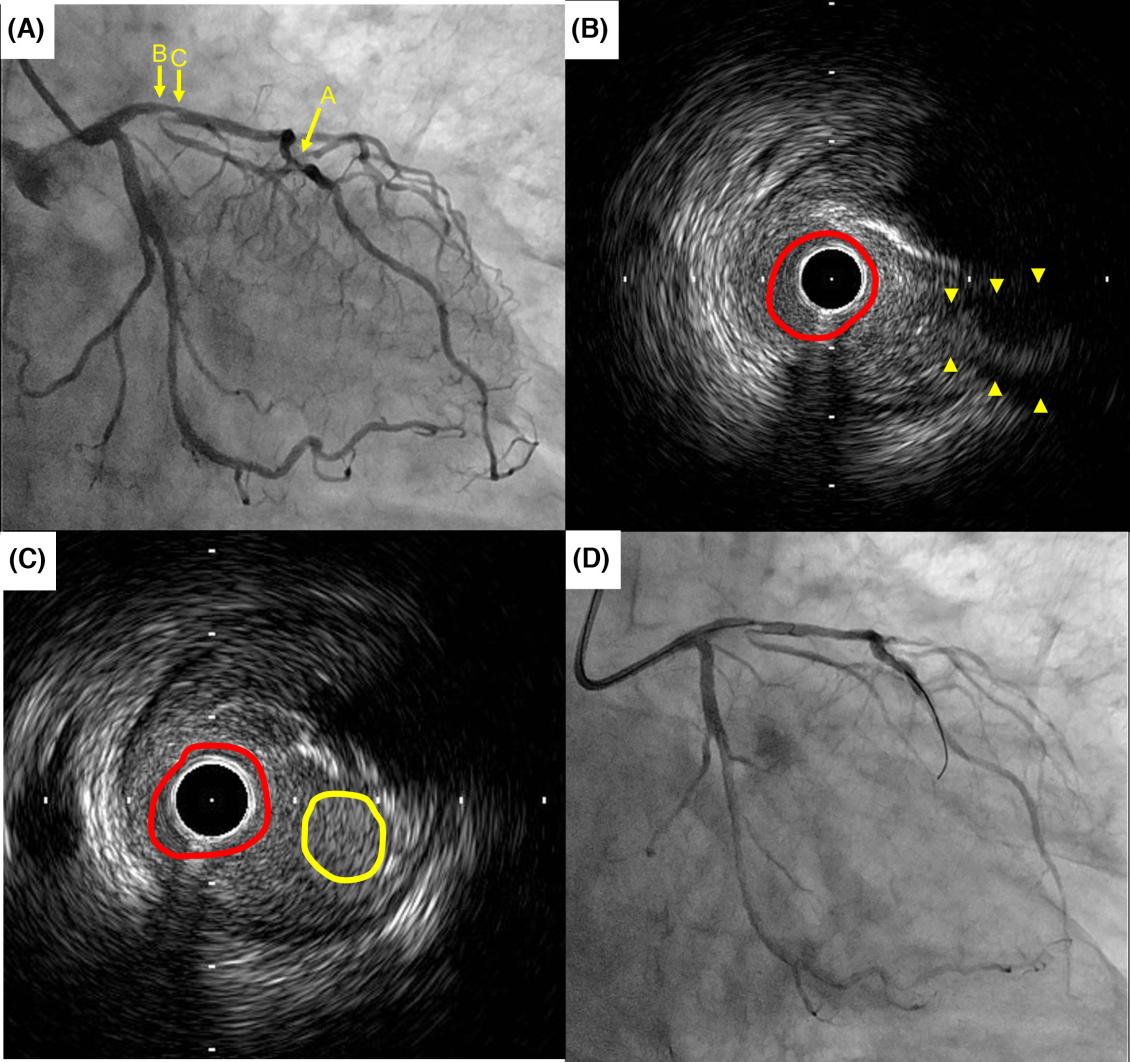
Fluoroscopic and IVUS images before atherectomy. (A) A coronary angiography. Arrow A indicates 75% stenosis at the mid‐LAD. Arrows B and C indicate the location of the IVUS images; (B) A cross‐sectional image of the ostium of the side branch (arrowheads). The circle indicates the lumen area; (C) A cross‐sectional image of the double lumen (circle). The red and yellow circles indicate the main lumen of the LAD and one leading to the side branch, respectively. (D) Failed reverse wire technique. IVUS = intravascular ultrasound, LAD = left anterior descending artery.
